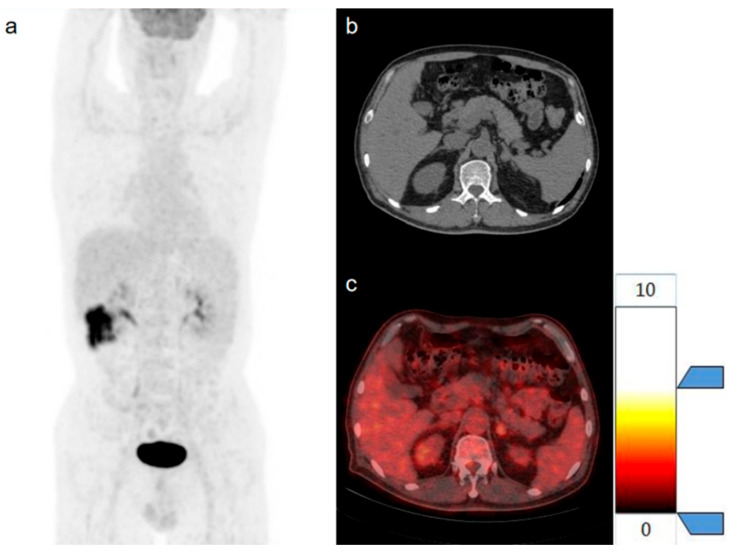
Patient No. 6 (a) The maximum density projection (MIP), (b) the high-resolution CT, and (c) the PET/CT.
